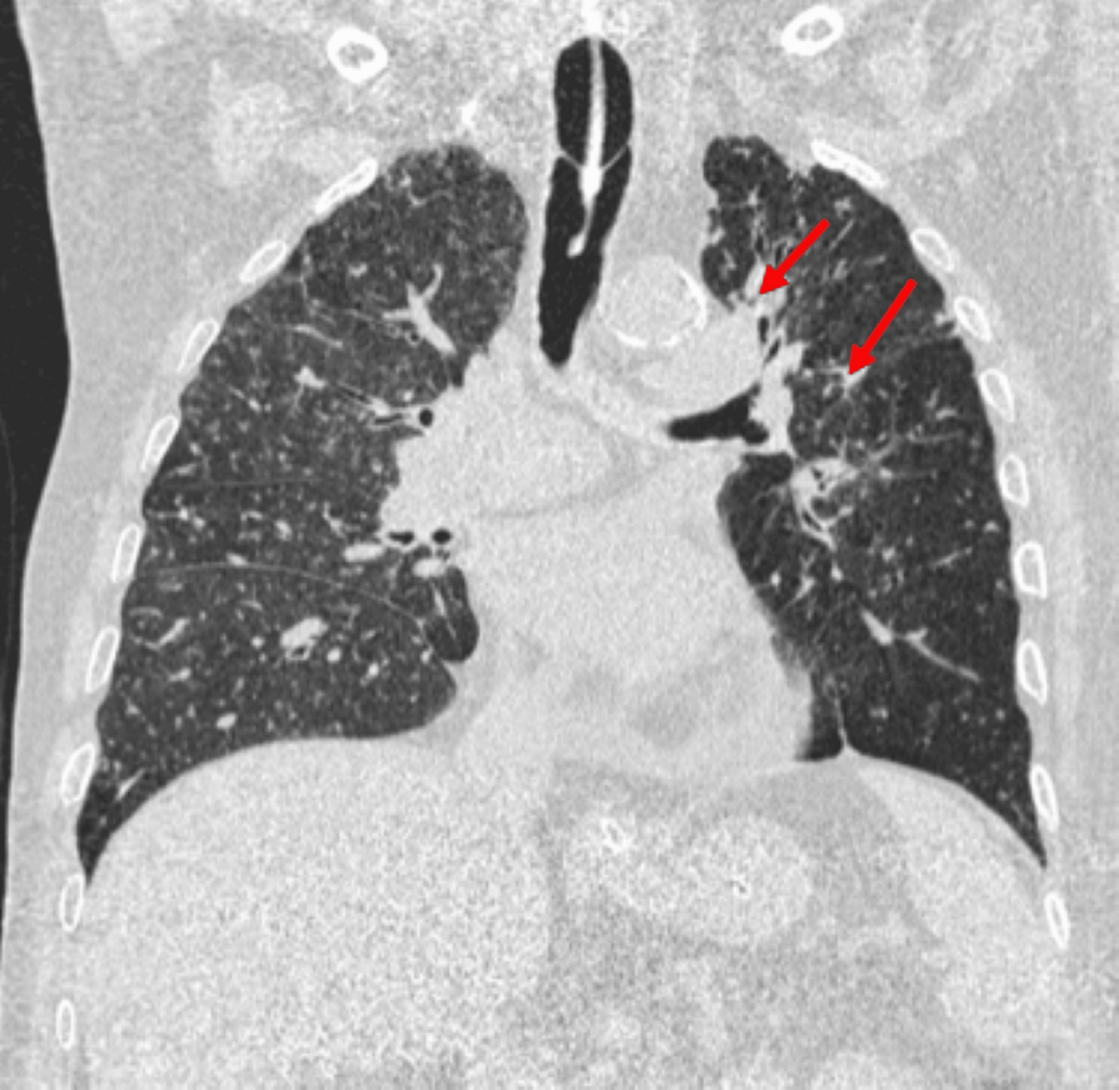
CT scan of the chest showing fibronodular changes (red arrow)
